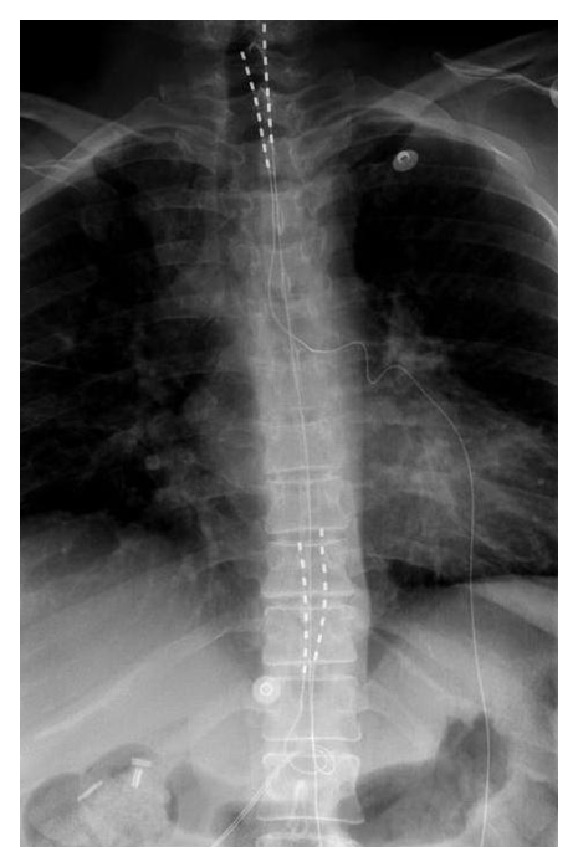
In this X-ray picture you will see the cervical and thoracic leads.
